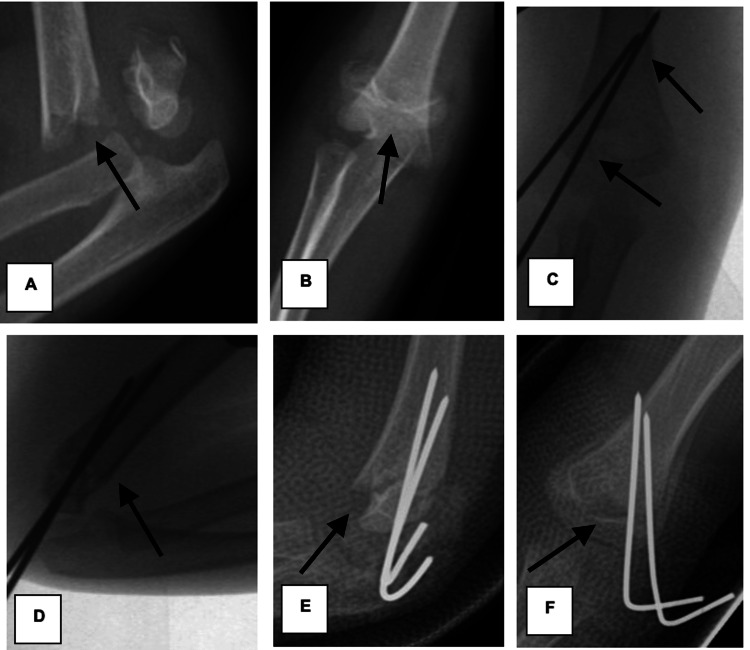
Inadequate fixation using lateral wires leading to postoperative loss of reduction from one of our cases (A, B) Preoperative AP and lateral radiographs of Gartland type III supracondylar fracture. (C, D) Intraoperative AP and lateral radiograph with 2 lateral wires showing adequate reduction but inadequate fixation (not bi-columnar, not divergent, separation of wires <1/3 circumference of the humerus at the fracture site). (E, F) Three weeks postoperative AP and lateral radiographs demonstrating loss of reduction. AP: anteroposterior
